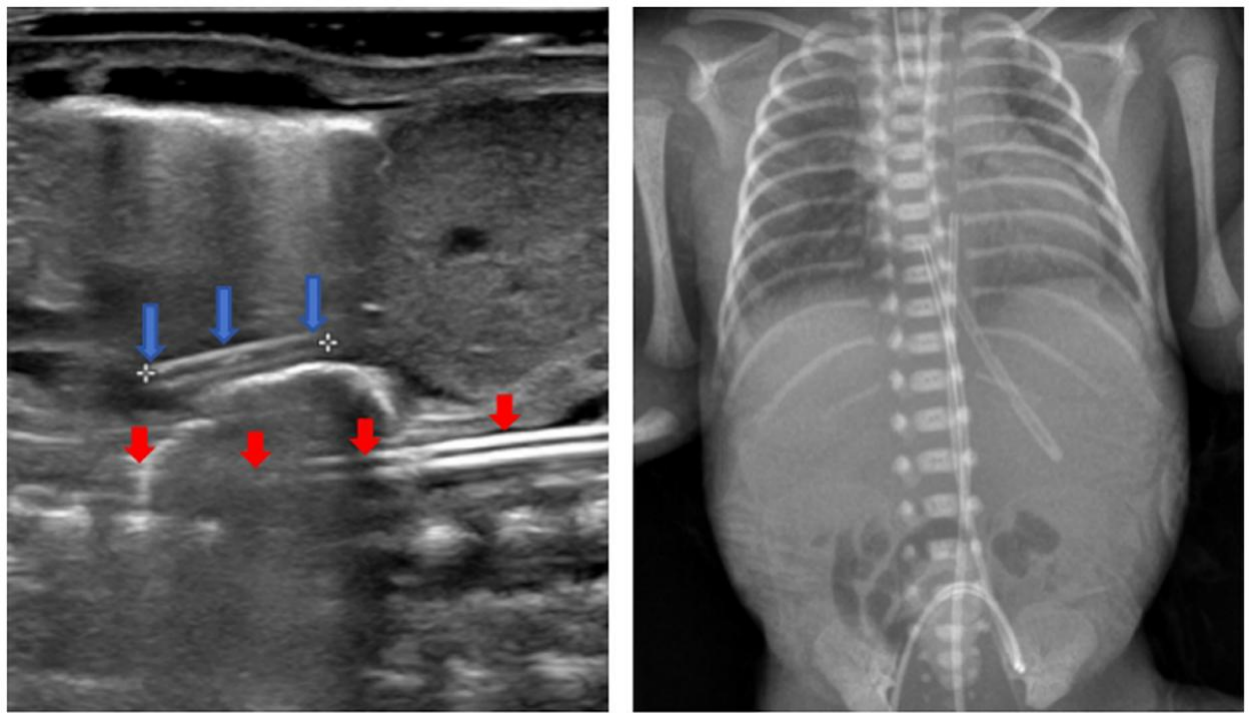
Point-of-care ultrasound (POCUS) confirmed the UVC tip approximately 1 cm above the diaphragm and and the UAC tip positioned at the mid-cardiac level. The corresponding radiograph demonstrated a UVC tip approximately 0.9 cm above the diaphragm and the UAC tip at the T7-T8 level. The endotracheal tube tip was positioned at the T3 vertebral level.The blue arrow in the image indicates the UVC, and the red arrow indicates the UAC.
